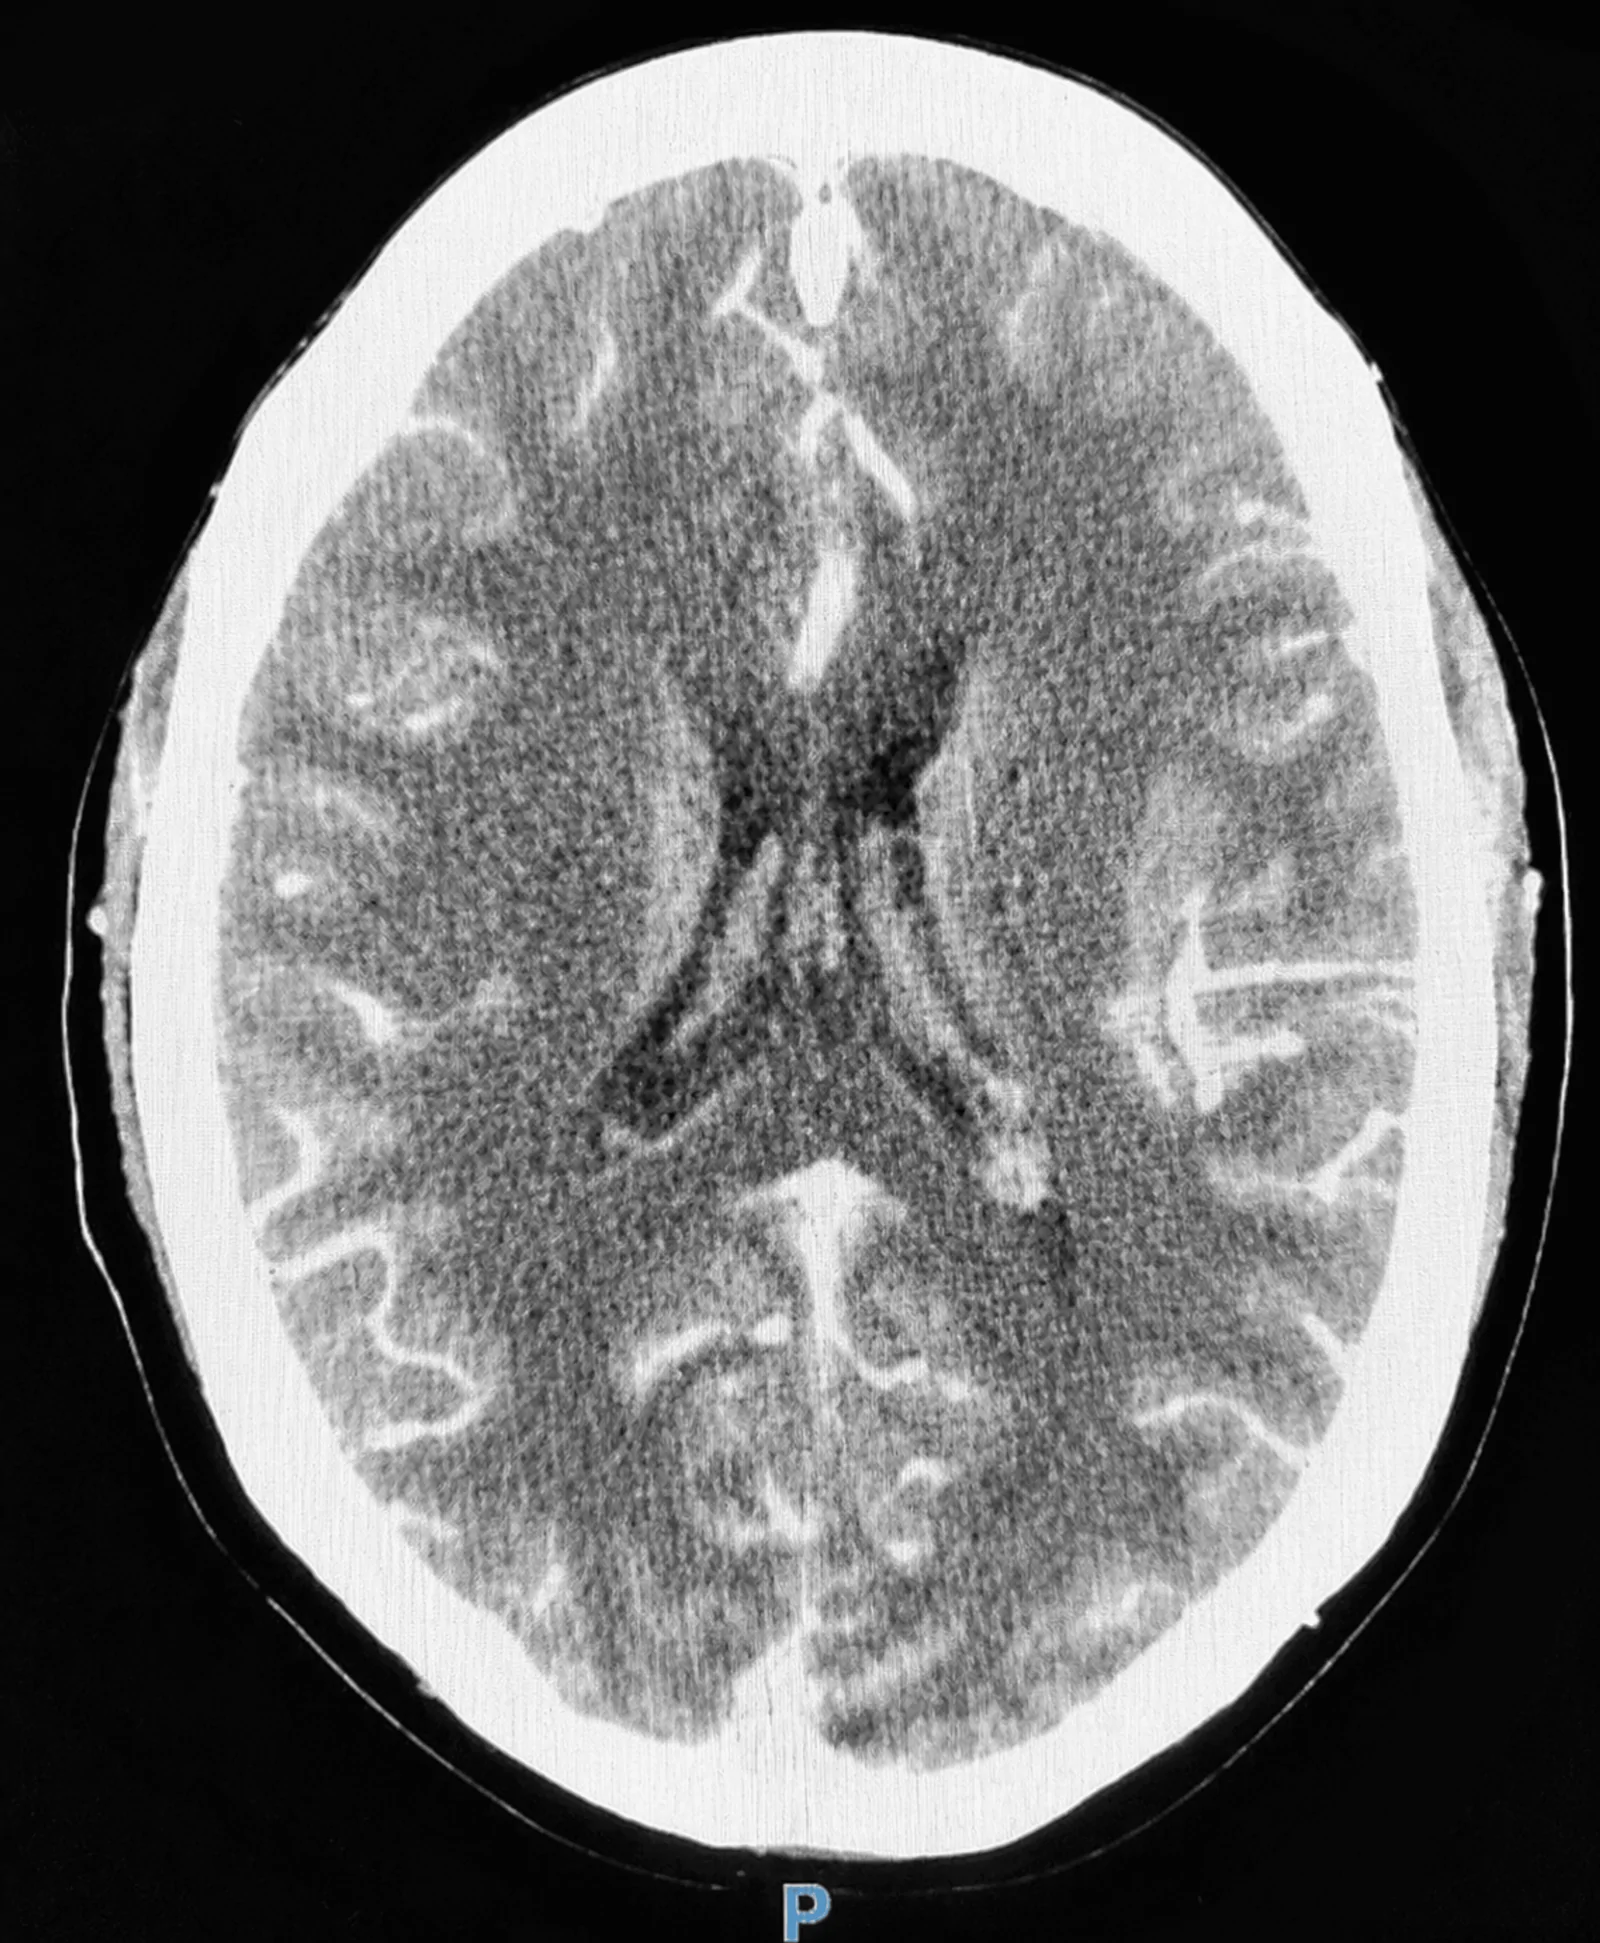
CT angiogram showing no intracranial aneurysm/arteriovenous malformation.
